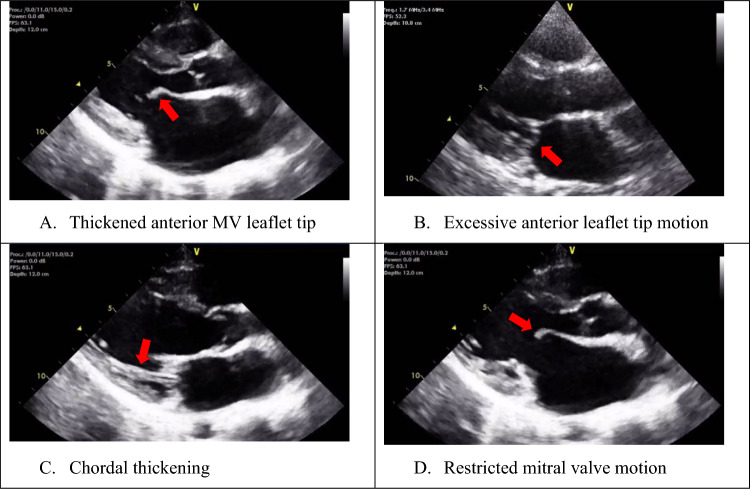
Mitral valve abnormalities associated with rheumatic heart disease. Parasternal long-axis views show A thickened anterior mitral valve leaflets (red arrow), B excessive anterior leaflet tip motion (red arrow), C chordal thickening (red arrow), and D restricted anterior leaflet tip motion (red arrow)
